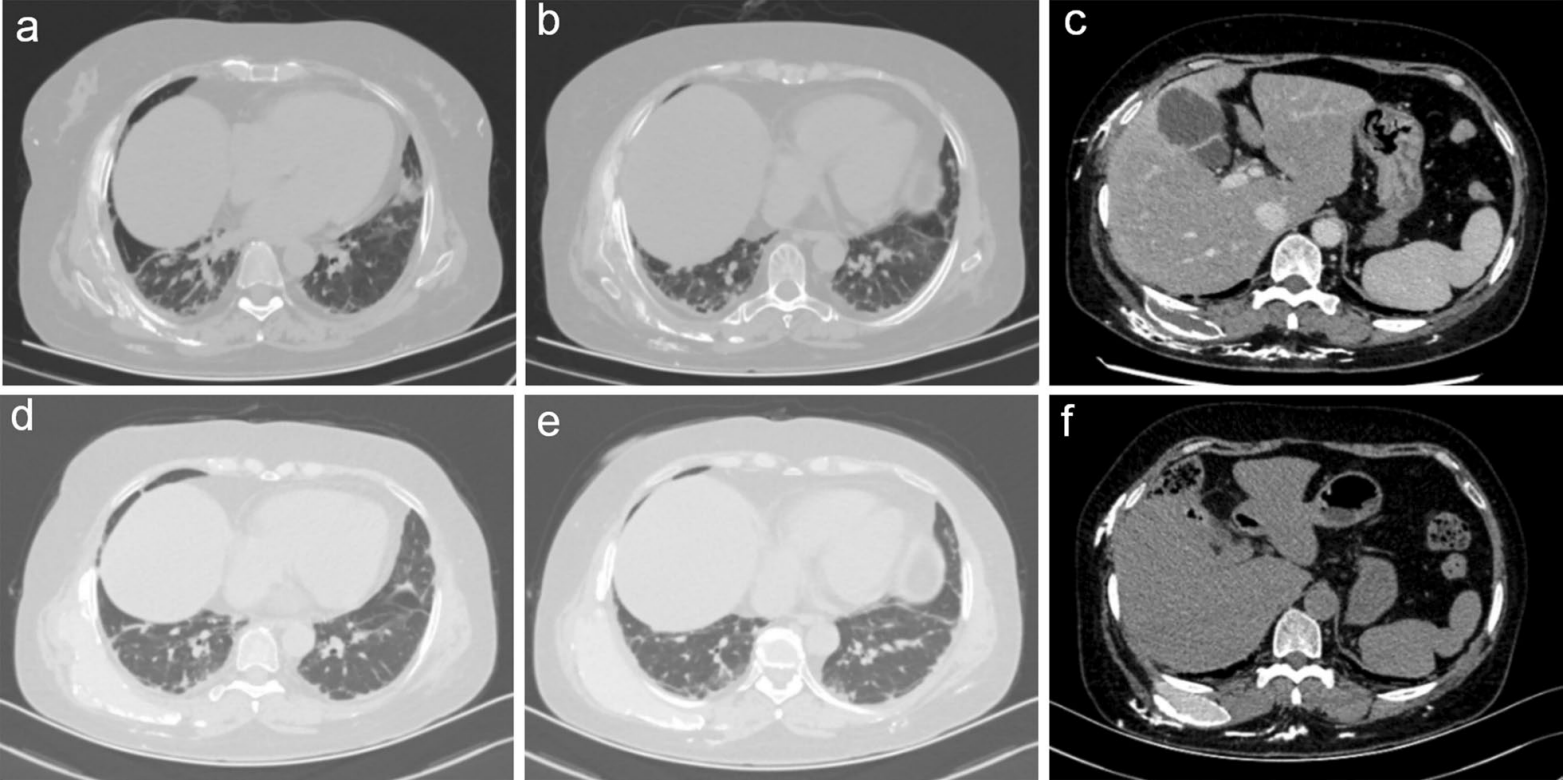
Chest computed tomography HRCT of the lung before (a, b, c) and after (d, e, f) treatment with tofacitinib for 6 months
